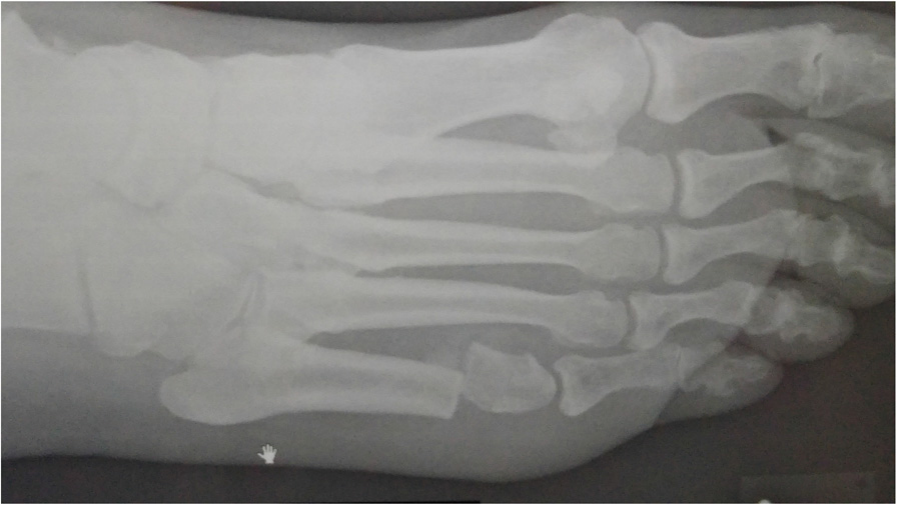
Oblique X-ray of patient in following figures, 1 month following surgery. The fifth MT head is significantly elevated and the osteotomy is healing
